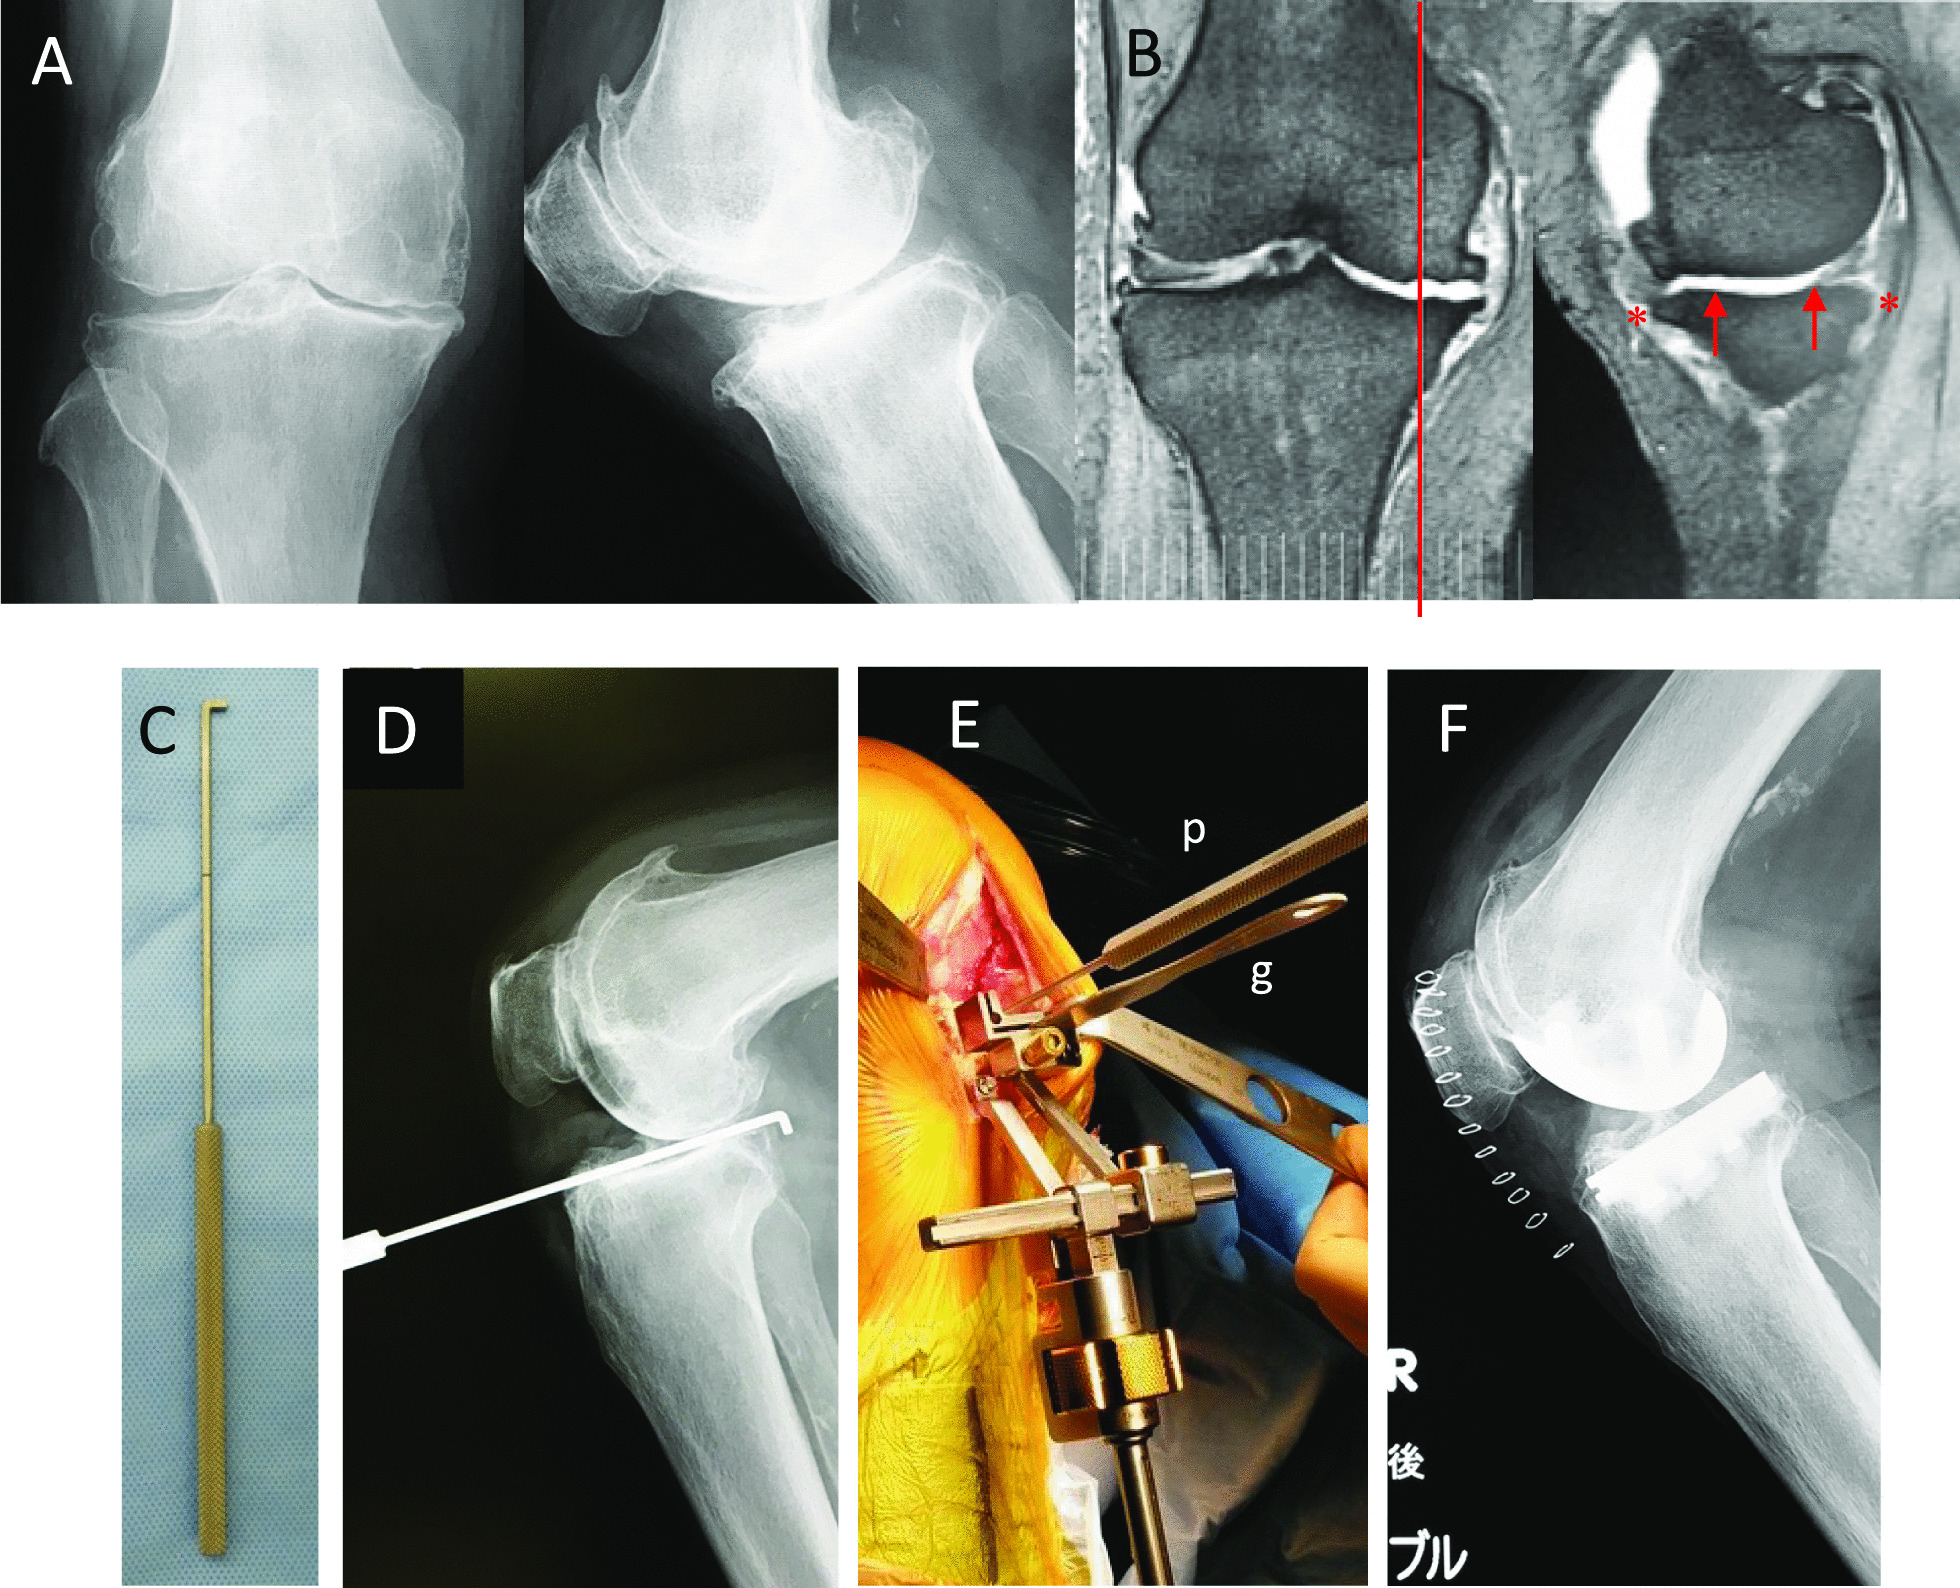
Case presentation. A Preoperative knee radiographs with Grade 4 OA. B Preoperative MRI. A red line: a slice line on the medial second quarter of the MTP. *: anterior and posterior “low” osteophyte. ↑: anterior and posterior “mild” depression on the MTP. C A hook probe. D An intraoperative lateral knee radiograph with the probe on the medial second quarter. E A photograph showing how to set the extramedullary cutting guide in this method. g: A gauge inserted into the slot of the tibial cut block. p: the probe. F Postoperative lateral knee radiograph. The native bony PTS is recreated
